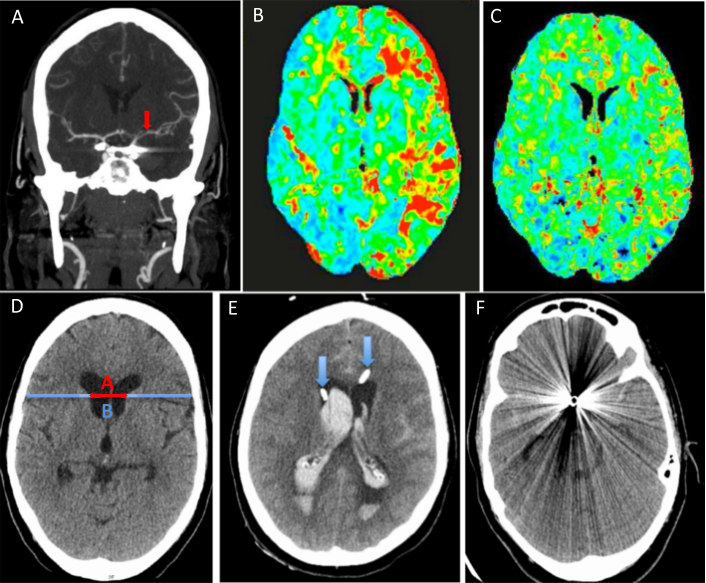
Multimodal computed tomography. (A) Computed tomography (CT) angiography (coronal plane) shows severe angiographic vasospasm in the left middle cerebral artery (MCA; red arrow). (B) CT perfusion displays increased mean transit time (increased shades of red in the left hemisphere) due to MCA vasospasm. (C) Same patient, CT perfusion repeated after 48 hours of haemodynamic augmentation, which shows resolution of perfusion changes. (D) Noncontrast computed tomography (NCCT) showing the measurement of bicaudate index (A/B) as proposed by van Gijn and colleagues [98]. (E) NCCT shows large volume of intraventricular haemorrhage. The tip of the bilateral external ventriculostomy drains can be seen in the anterior horn of the lateral ventricles (blue arrows). (F) NCCT quality severely compromised by artefacts generated by the metallic colis.
